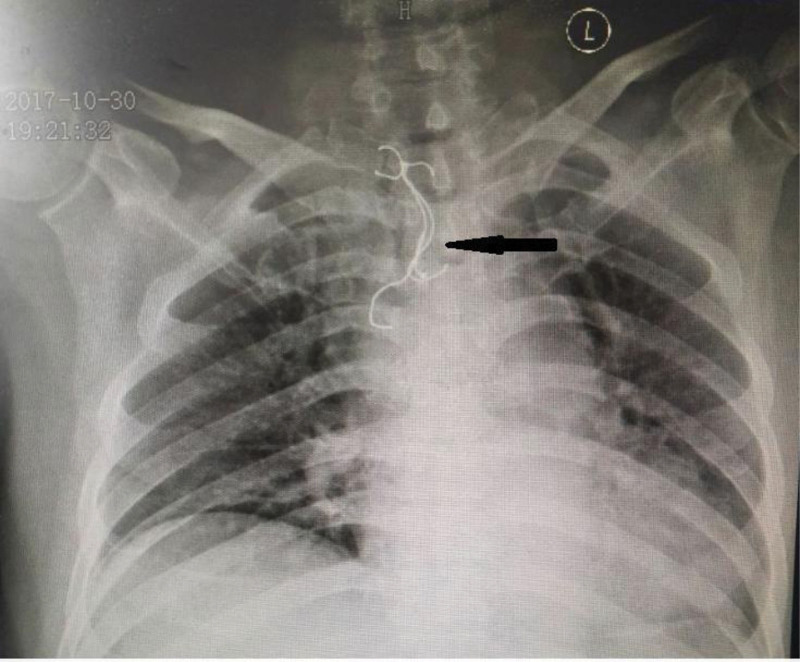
Chest radiography—arrow pointing towards the large denture.
